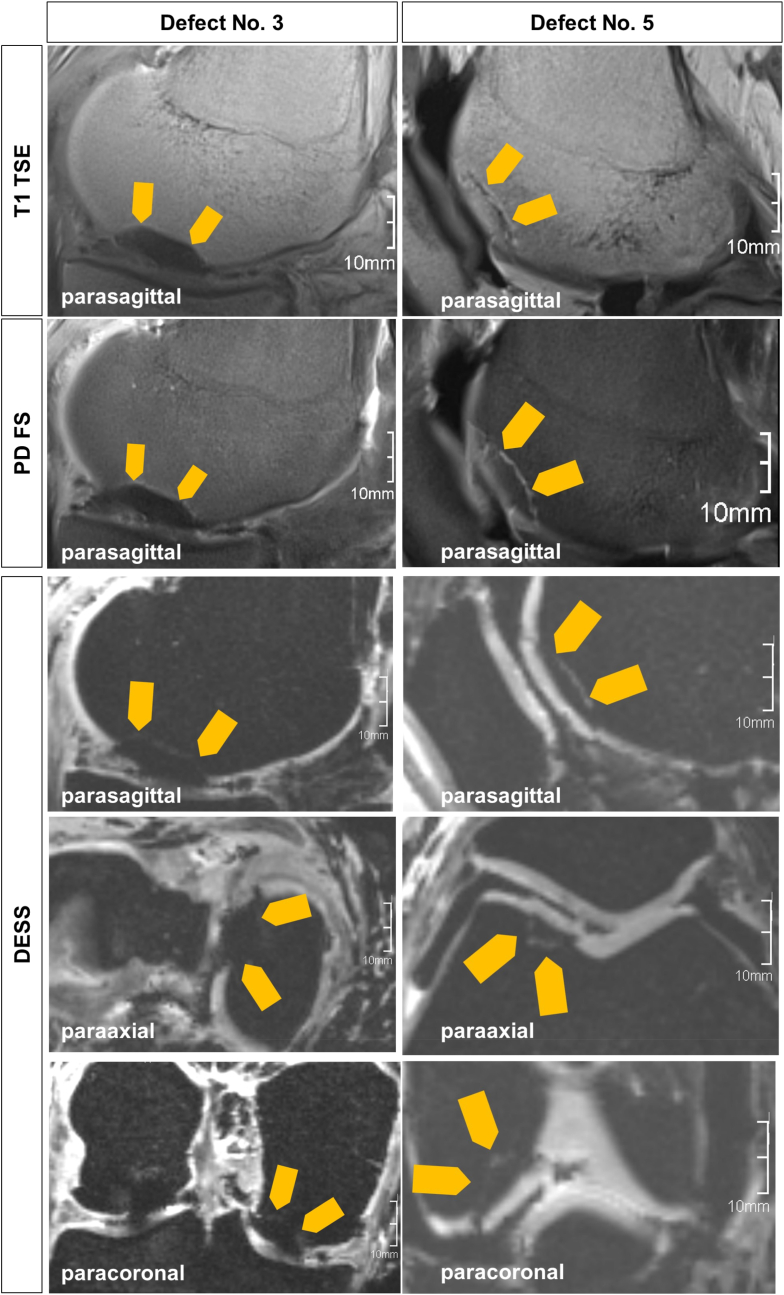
Exemplary imaging of the empty defect No. 3 (K2) and defect No. 5 (K3) with remaining osteochondral fragment in the MRI. T1 turbo spin echo (TSE) sequence, proton density fat saturation (PD FS), double-echo steady-state (DESS). The orange arrows mark the defect. (For interpretation of the references to colour in this figure legend, the reader is referred to the Web version of this article.)
